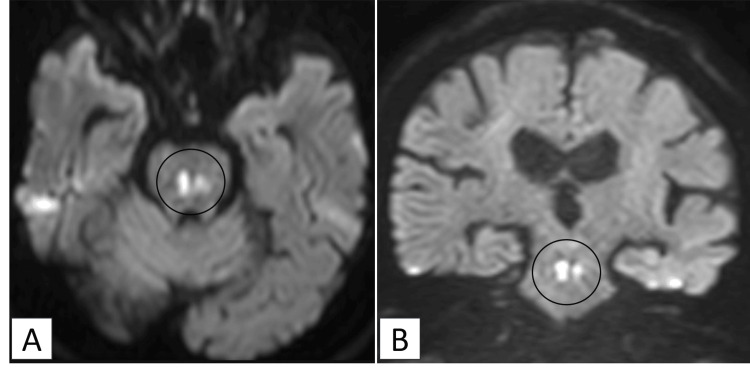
Case 2: Diffusion-weighted images (DWI) on day seven The axial view (A) and coronal view (B) both demonstrate a distinct, point-like hyperintense lesion in the central pons (circles).
